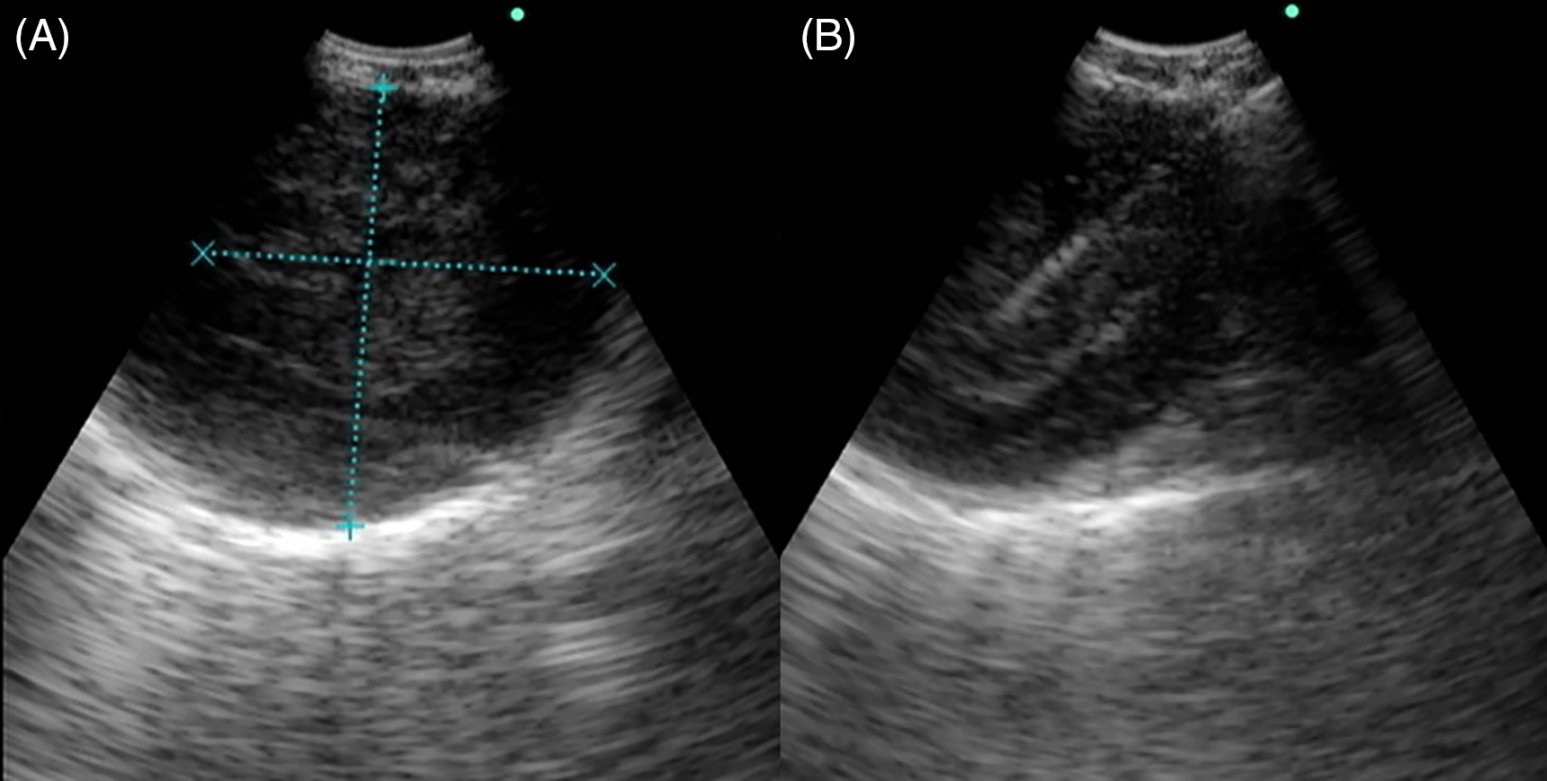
Endobronchial ultrasound (EBUS). (A, B) EBUS shows a well‐defined hypoechoic mass with posterior acoustic enhancement.
